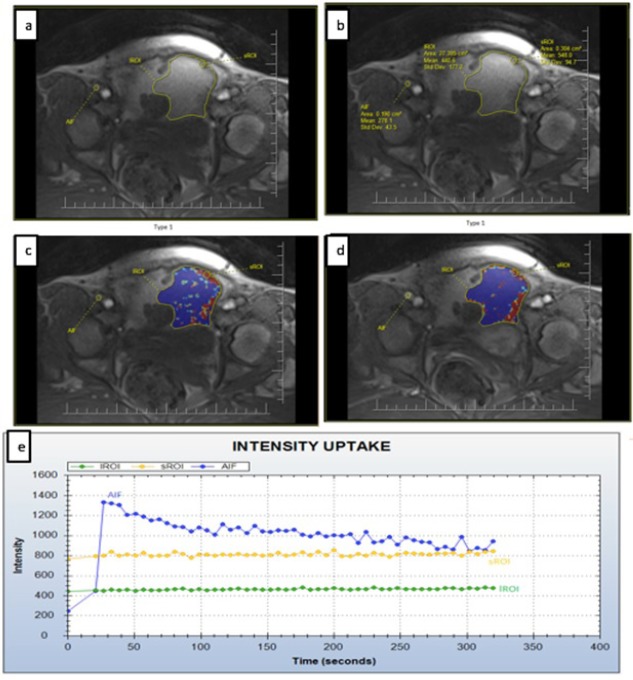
Type I Intensity Curve Along with the Corresponding Quantitative Measurements on the Patient’s MRI; (a) Source; (b) Details of the source; (c) Kep; (d) Ktrans; (e) TIC
